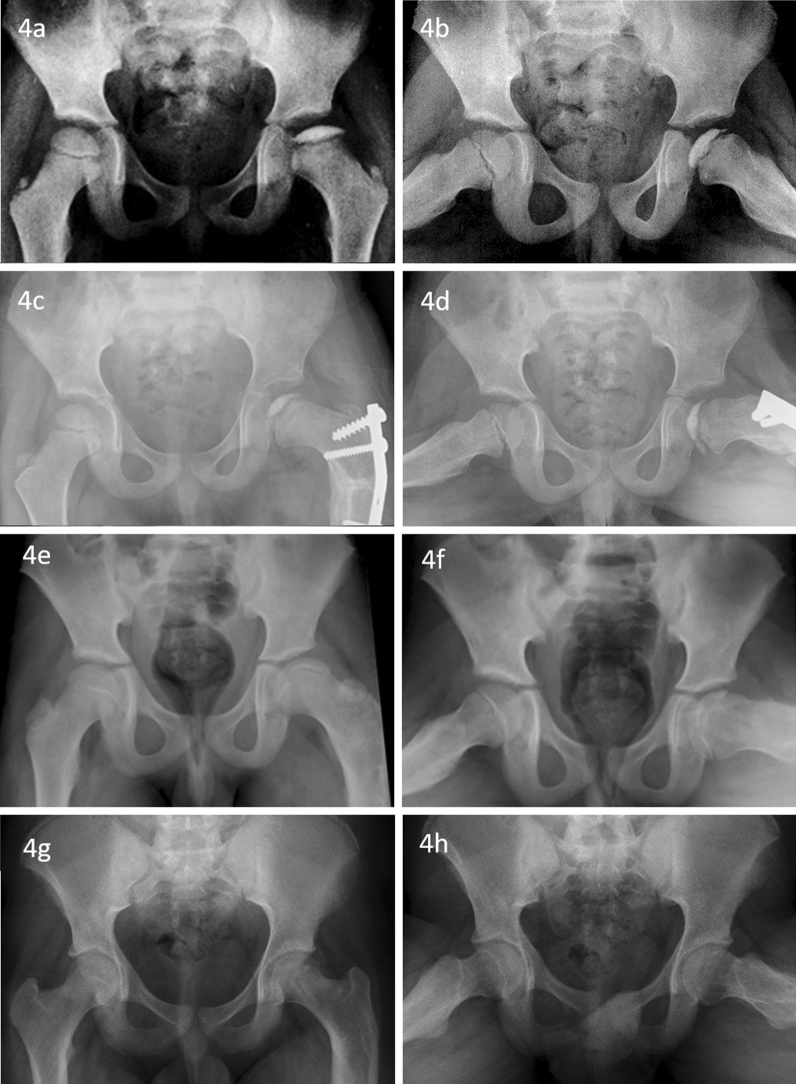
Anteroposterior (a) and frog-lateral (b) radiographs of a boy with the age of onset 5.5 years with left side Perthes disease (a, b). The Reimer’s index was 31.75 % and difference was 12.29 % (a). He underwent varus derotation osteotomy with trochanteric epiphysiodesis (c, d). The radiographs at healing (e, f) and skeletal maturity (g, h) showed good outcome
